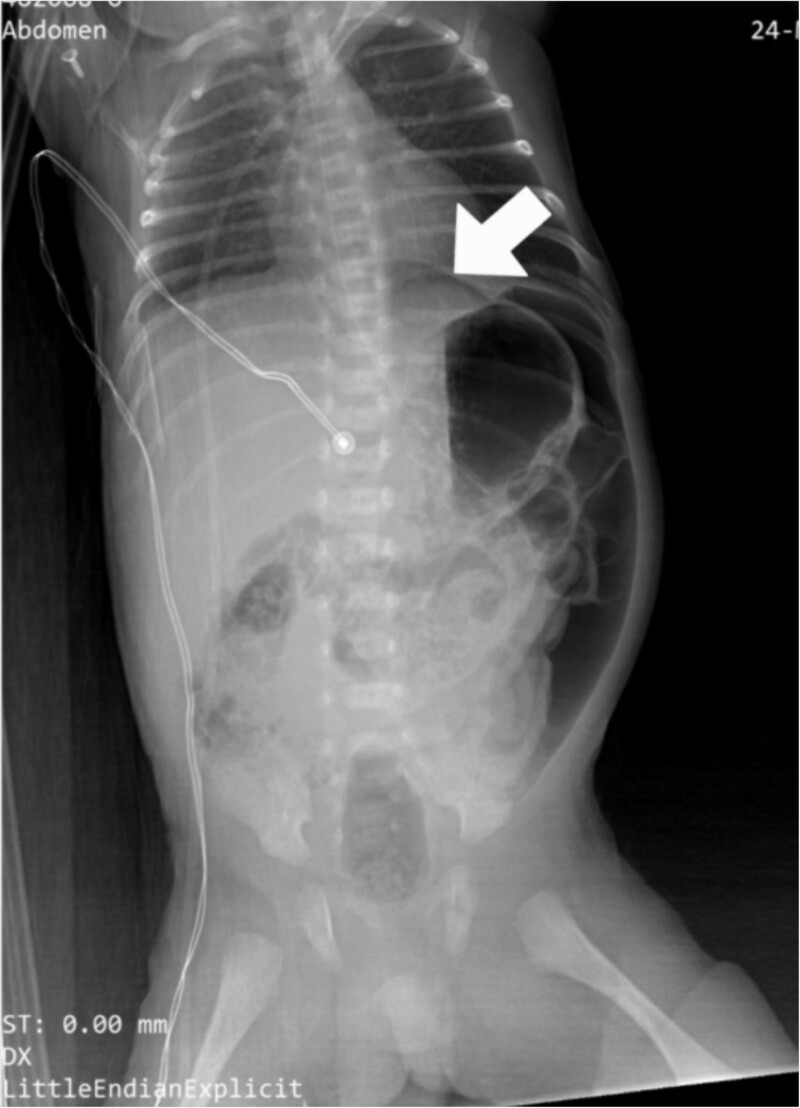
Preoperative abdominal X-ray: lateral decubitus view showing pneumoperitoneum (white arrows).
